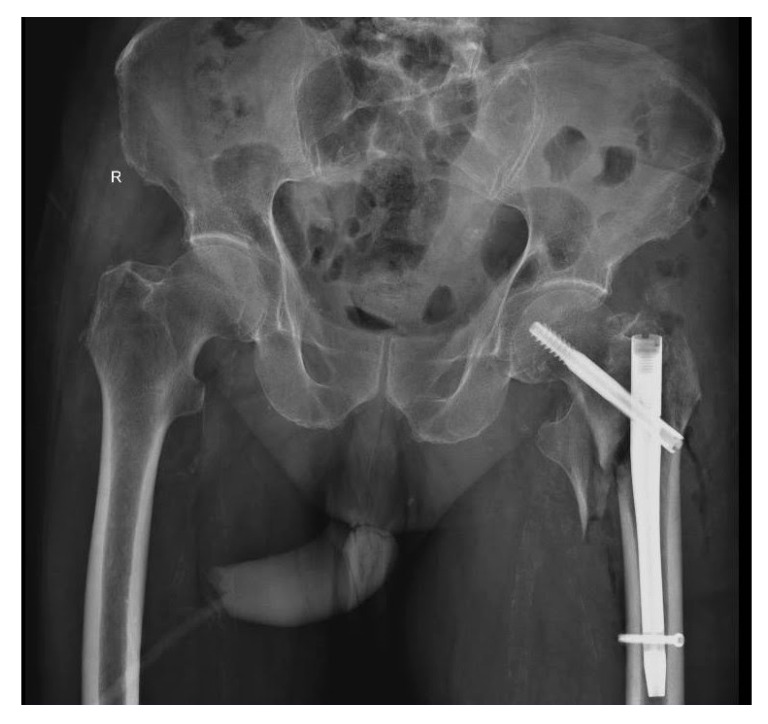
Post-surgery pelvic X-ray, anteroposterior incidence. Description: male subject, urinary catheter. Right hip with minimal narrowing of the joint space. Left hip with osteosynthesis material (intramedullary rod) in the proximal segment of the femur with distal nailing. Bone fragment with dorsal and internal displacement. Coxo-femural spaces maintained. Acetabular surfaces with sclerosis.
